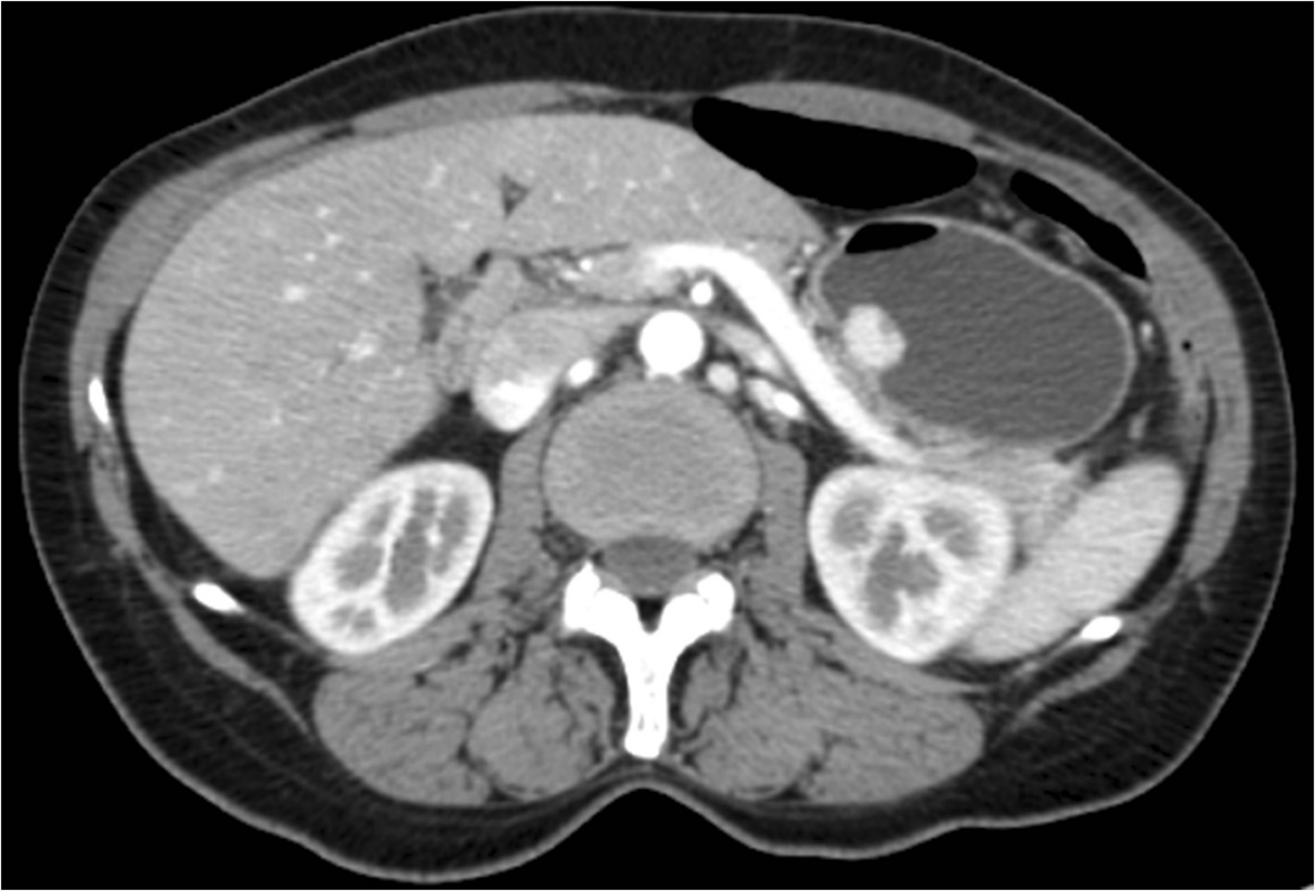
Abdomen computed tomography scan showed a gastric polyp with arterial enhancement and an apical area of necrosis.
